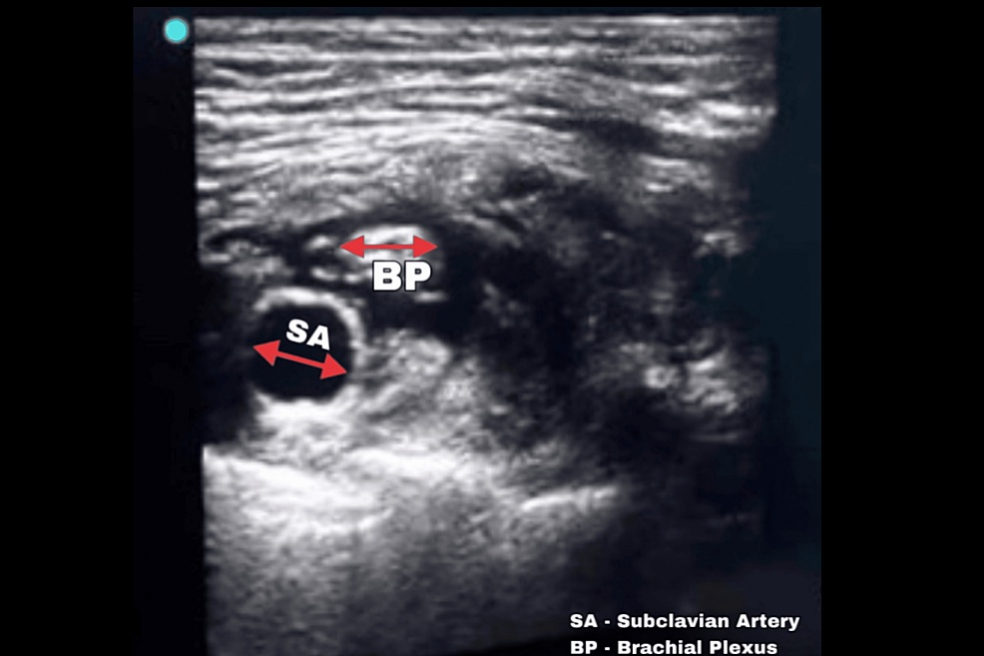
The ultrasound image depicting the post-supraclavicular local anesthetic spread around the brachial plexus.
